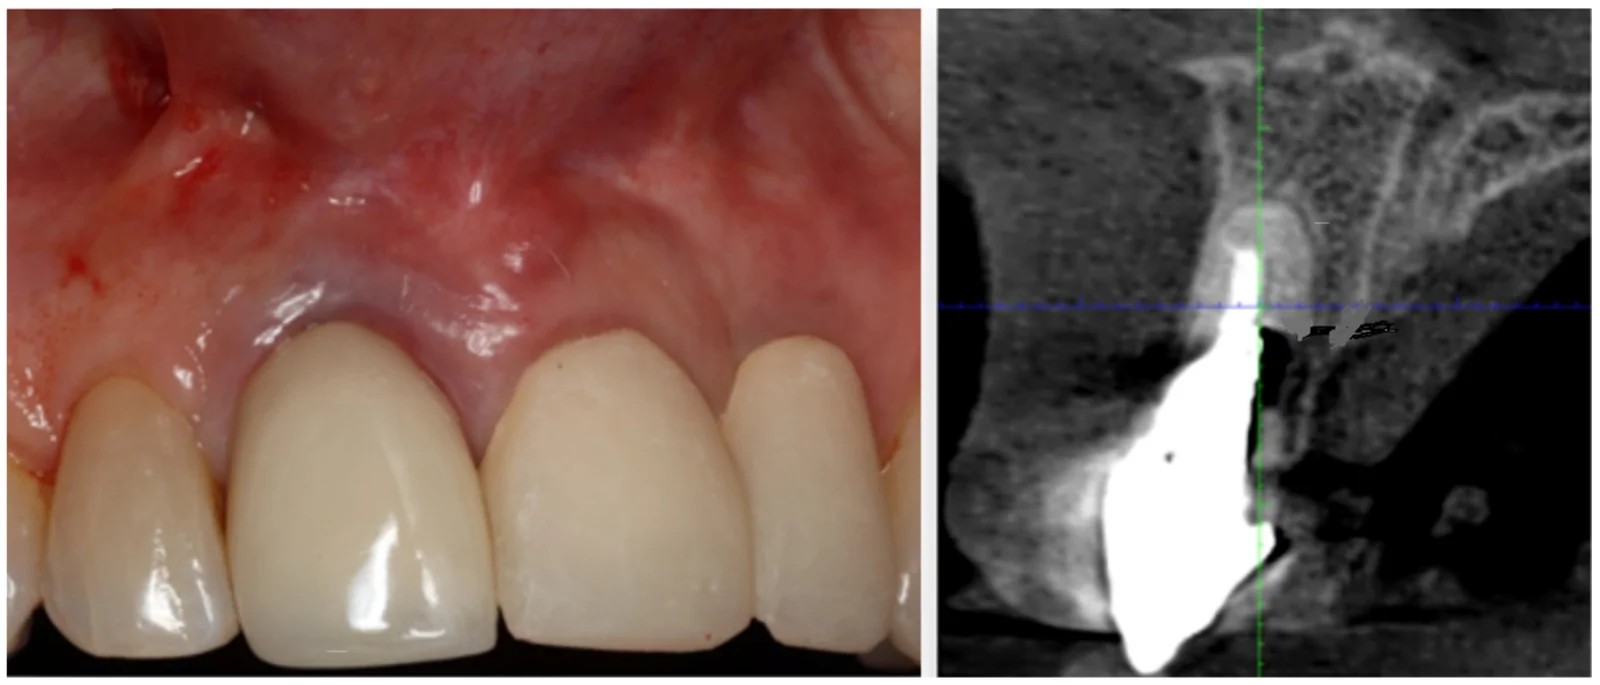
Initial clinical presentation demonstrating discoloration and soft-tissue shadowing associated with the maxillary right central incisor (left). CBCT cross-sectional image demonstrating extensive buccal plate loss and root dehiscence (right).
